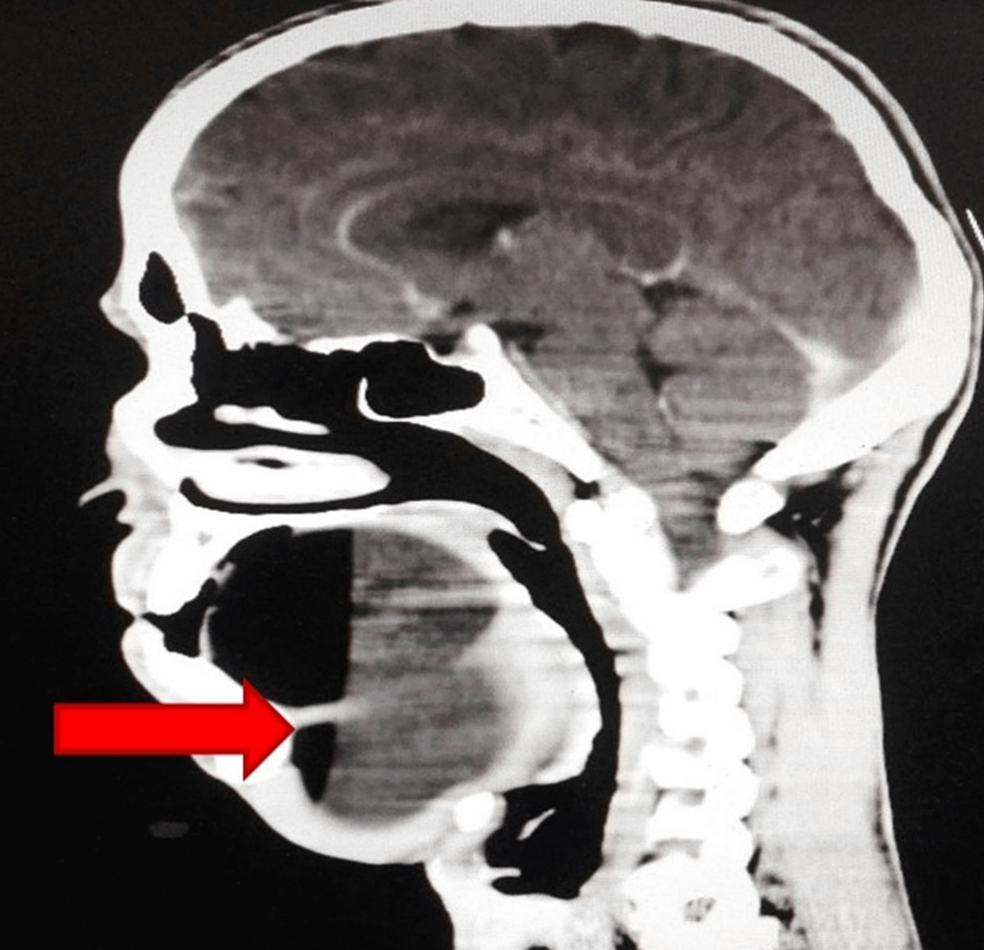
CT scan sagittal view showing non-enhancing mass in the floor of mouth
